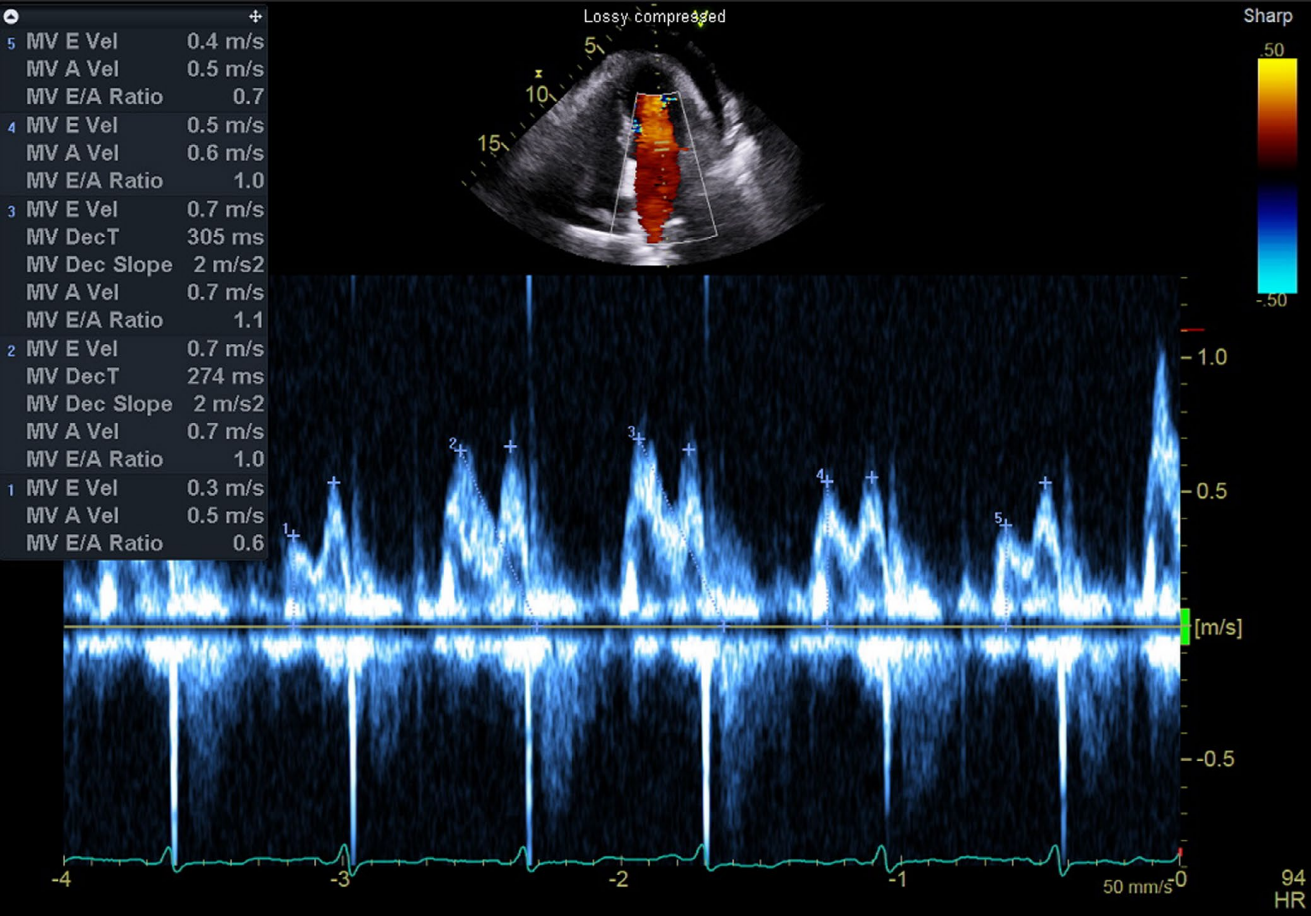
Pulsed Doppler echocardiography of blood entering the left ventricle from the left atrium, acquired from the apical four‐chamber position. Each pair of spikes represents inflow during diastole, the preceding representing early passive diastolic filling, the later contributed by atrial contraction. Between the second and the sixth complexes, early‐diastolic mitral inflow velocities measure 0.3, 0.7, 0.7, 0.5, to 0.4 m/s, respectively
